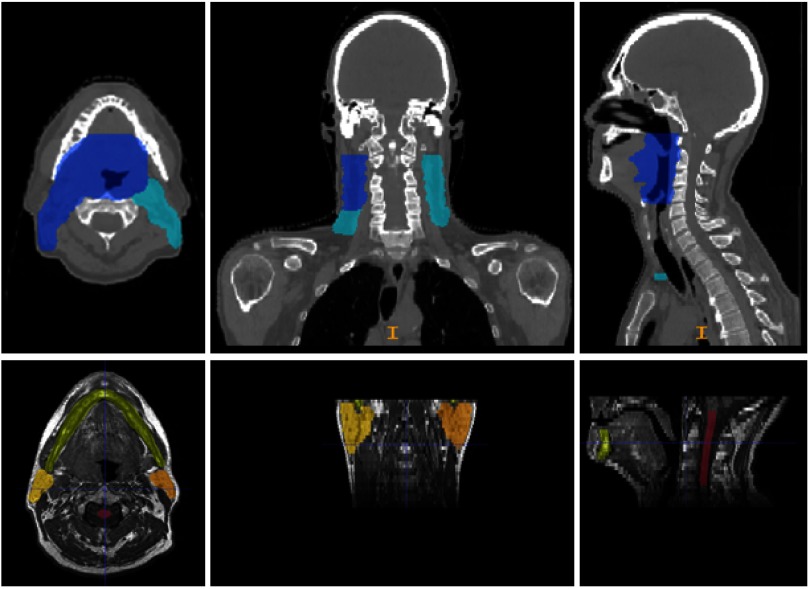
This figure depicts axial, coronal and sagittal slices of the CT (top
